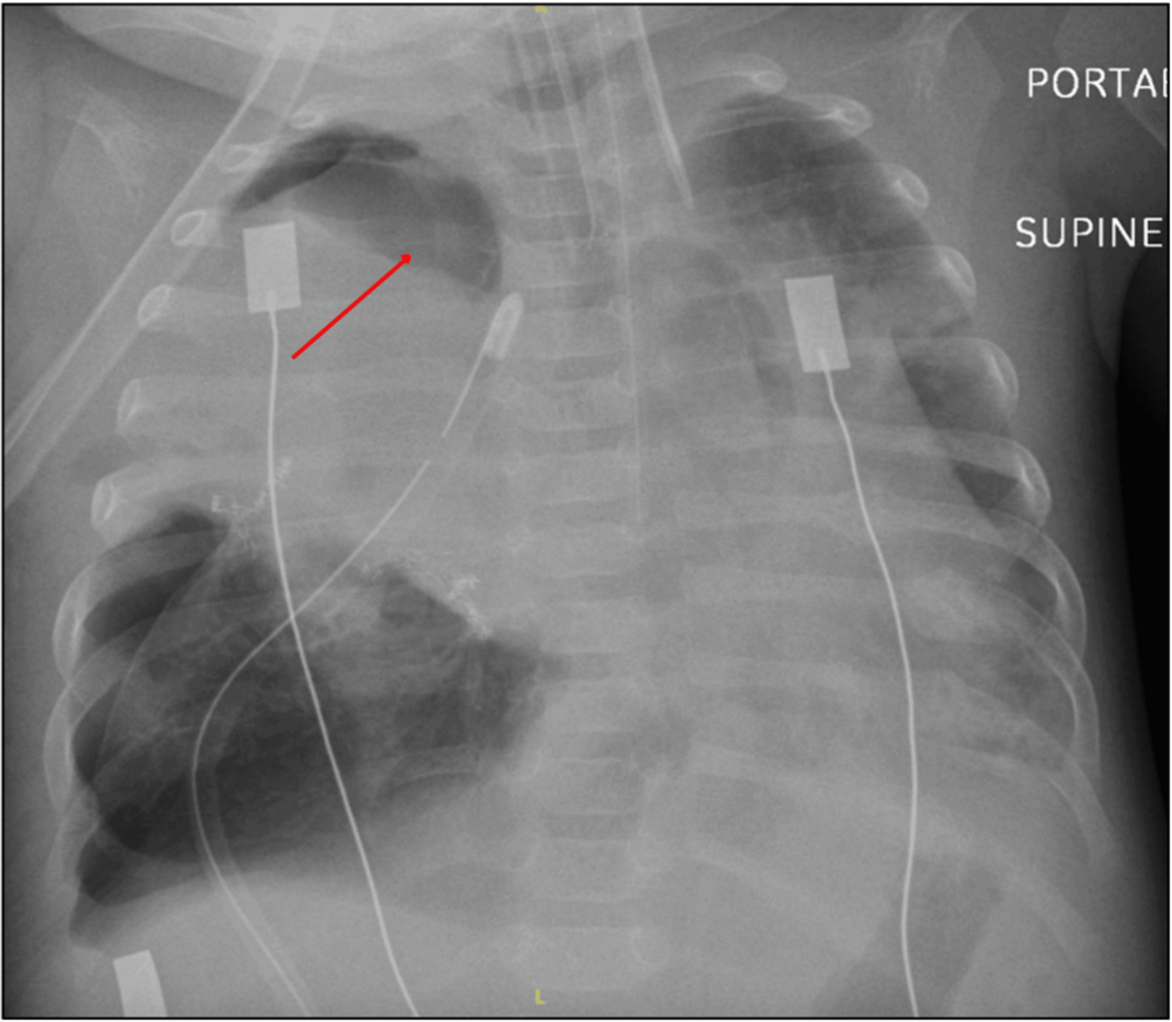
Anteroposterior chest radiograph on postoperative day 3 following right middle lobectomy The red arrow indicates the re-expanded residual right lung with resolution of the mediastinal shift; chest drains remain in situ.
